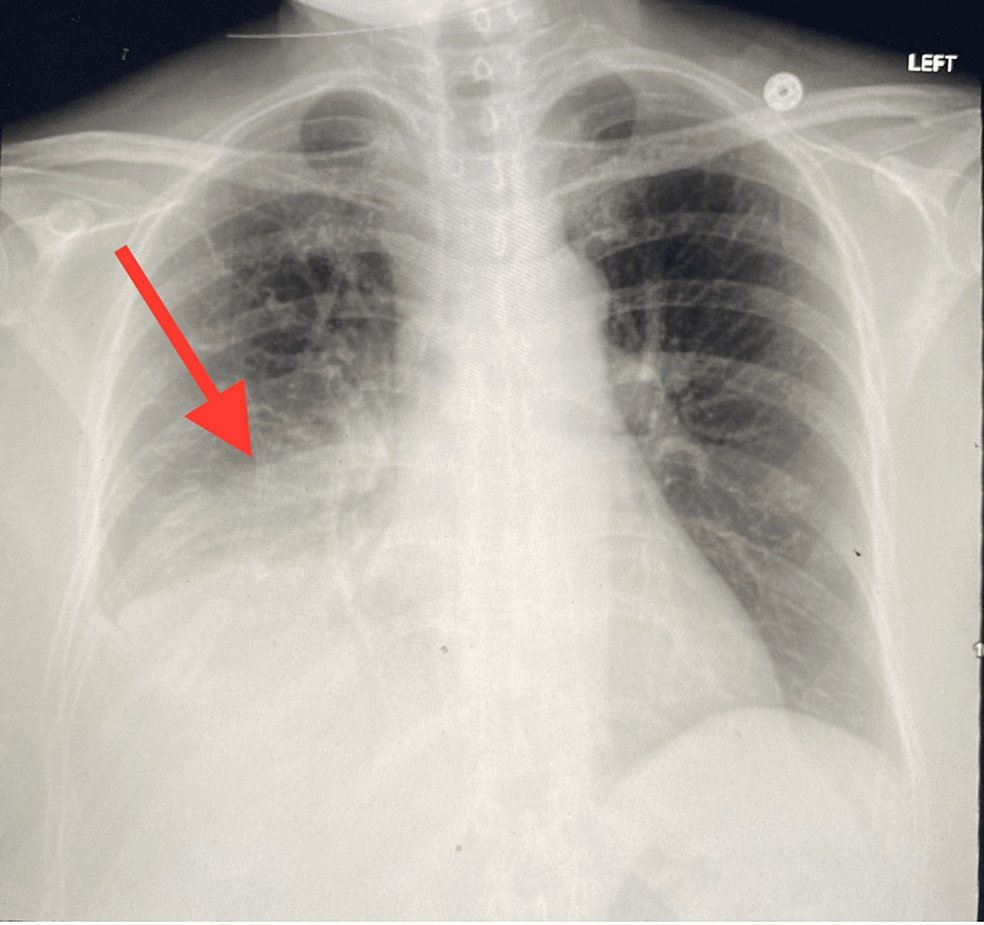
Chest x-ray showing airspace consolidations in the right lower lobe concerning for pneumonia.
